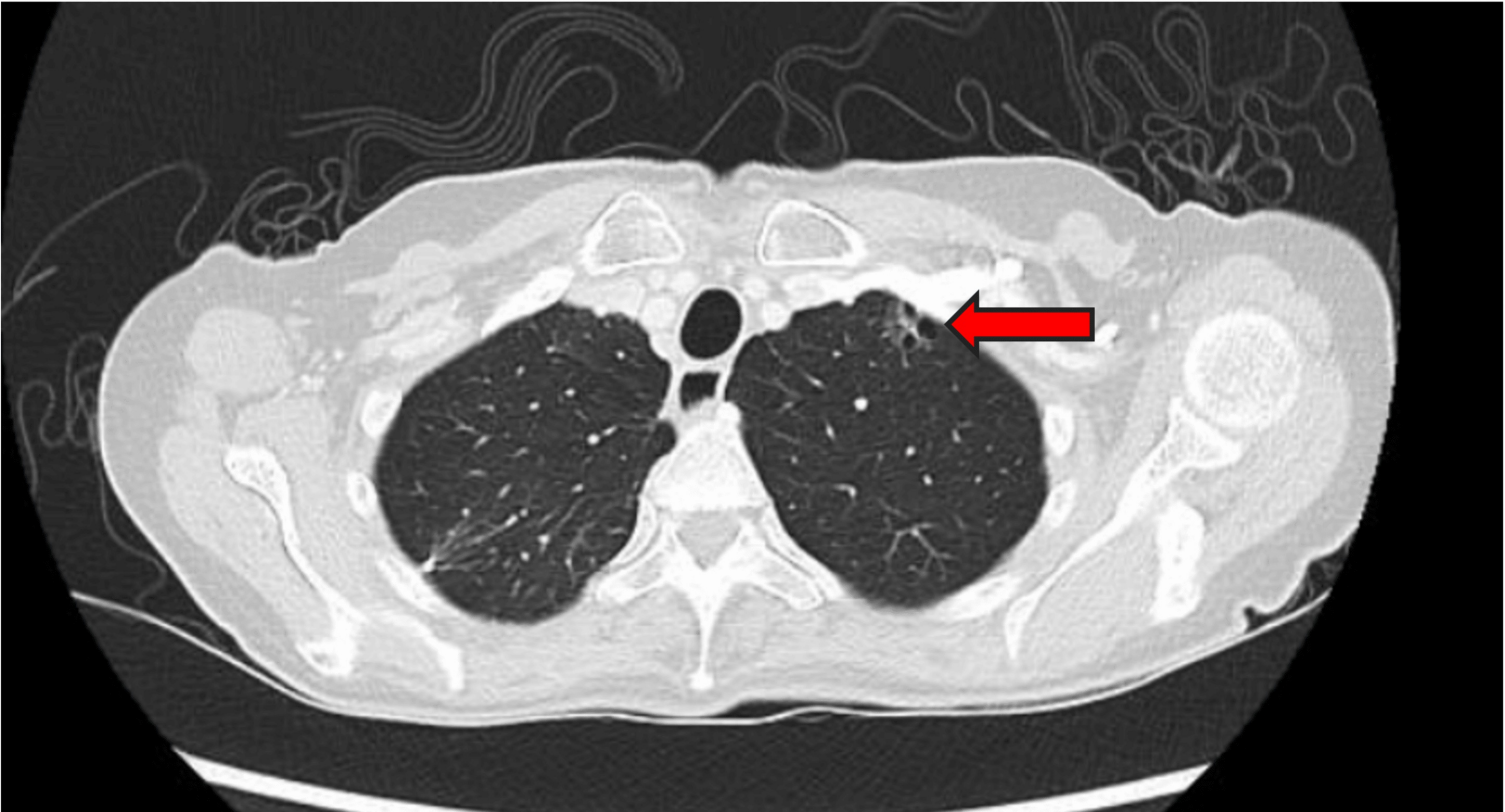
Axial cut of the chest CT showing a new 1 cm cystic focus at the left lung apex (red arrow). This occurred a year after the patient had a wedge resection of the right upper lobe pulmonary metastasis. CT: computed tomography
